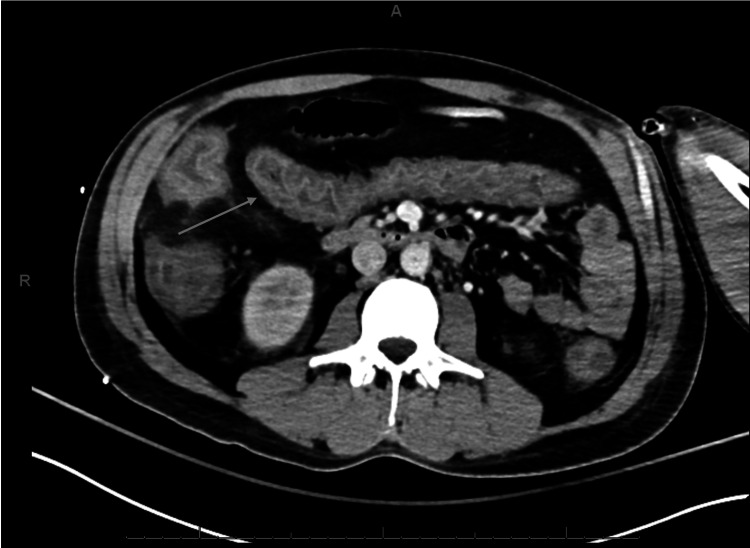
CT abdomen and pelvis with contrast, axial plane showing diffuse colonic wall thickening
